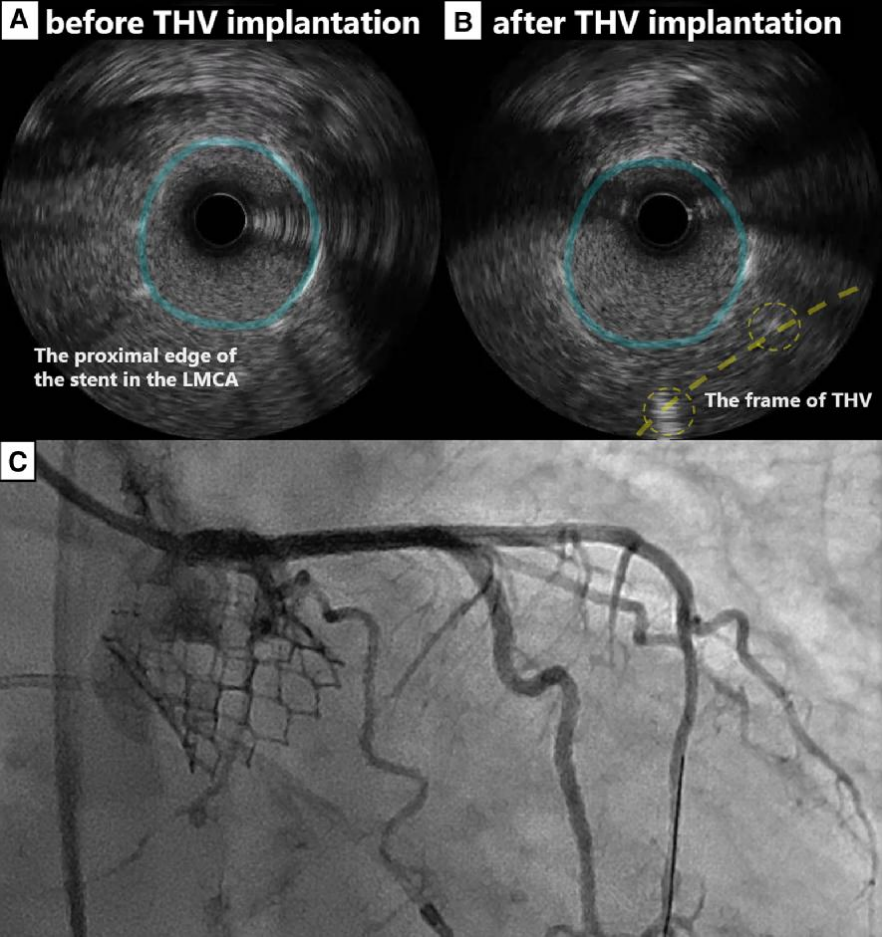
The intravascular ultrasound findings of the ostium of the stent in the left main coronary artery before (*A*) and after (*B*) the transcatheter heart valve was implanted, and coronary angiography (*C*): the stent (solid lines) in the left main coronary artery was not deformed. In (*B*), the frame of the transcatheter heart valve (dashed lines) was proximal to the stent in the left main coronary artery. IVUS, intravascular ultrasound, LMCA, left main coronary artery, THV, transcatheter heart valve.
